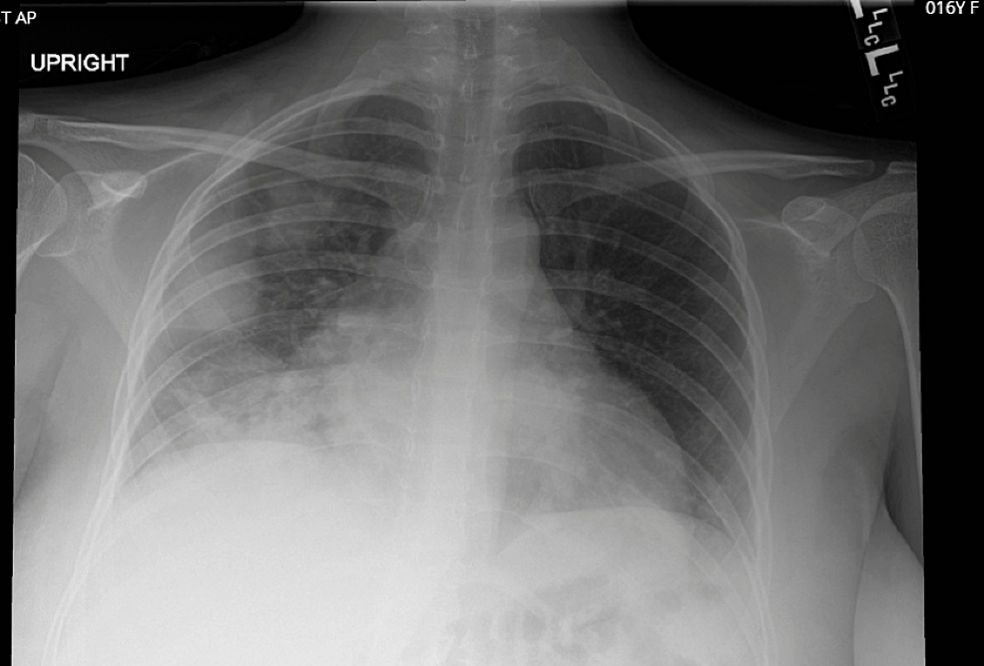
Chest X-Ray demonstrating patchy pulmonary opacities throughout the lungs, right greater than left.
